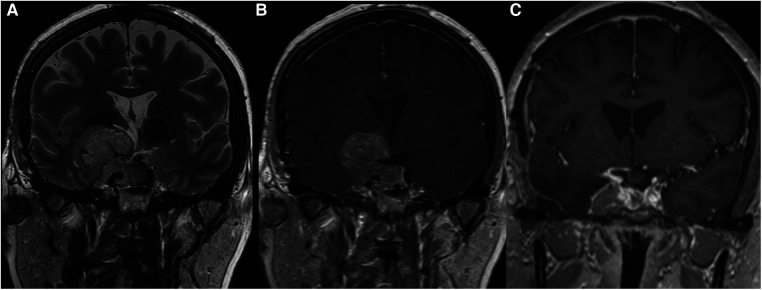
A giant macroadenoma showed invasion of the right cavernous sinus (Knosp 4), and it was classified as grade 4 according to the relationship with the diaphragm (Pictures A and B showing a coronal T2- and T1-weighted MRI after gadolinium administration, respectively). A combined approach through the use of a pterional approach and an endoscopic endonasal approach allowed to obtain a partial resection after addressing respectively the portion in the subarachnoid space and the sellar and suprasellar portion. The residual tumor in the right cavernous sinus (Picture C) was treated through Gamma Knife irradiation, and the tumor remained stable at 5 years of follow-up.
